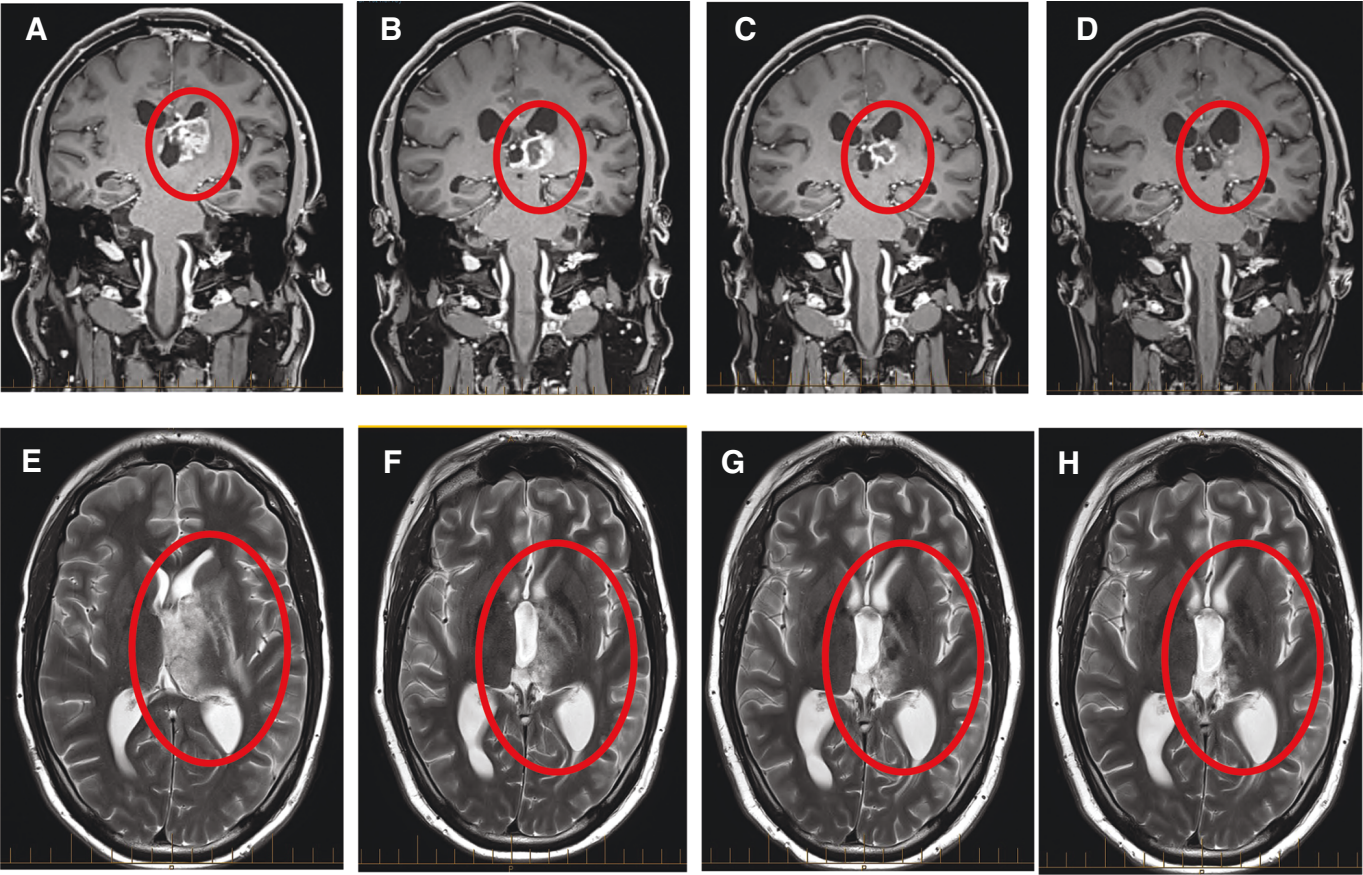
Radiologic response to PARP inhibition in a diffuse midline glioma (DMG) patient with pathogenic germline variants in *BRCA2* and *FANCE.* T1-weighted MRI brain with contrast in a patient with relapsed DMG, germline *BRCA2,* and *FANCE* pathogenic variants, following treatment with a PARP inhibitor and durvalumab at (A) pretreatment, (B) 6 weeks, (C) 2.5 months and (D) 5 months after commencement of therapy. Axial non-contrast T2-weighted images show the same time points in panels E, F, G, and H, respectively.
